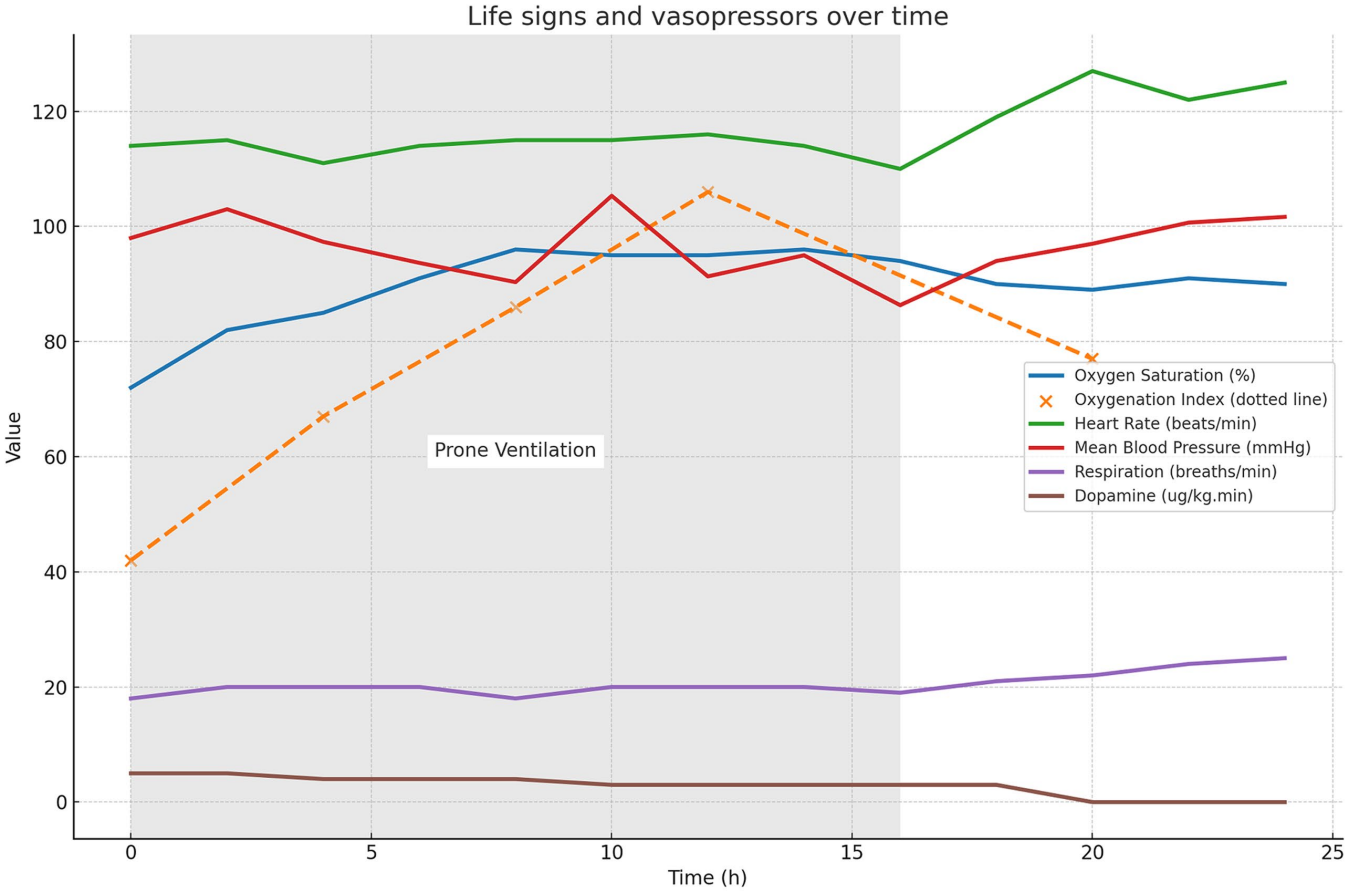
Chest computed tomography angiography (CTA) reveals embolization of both the main trunk of the right pulmonary artery and multiple arterial branches bilaterally.
